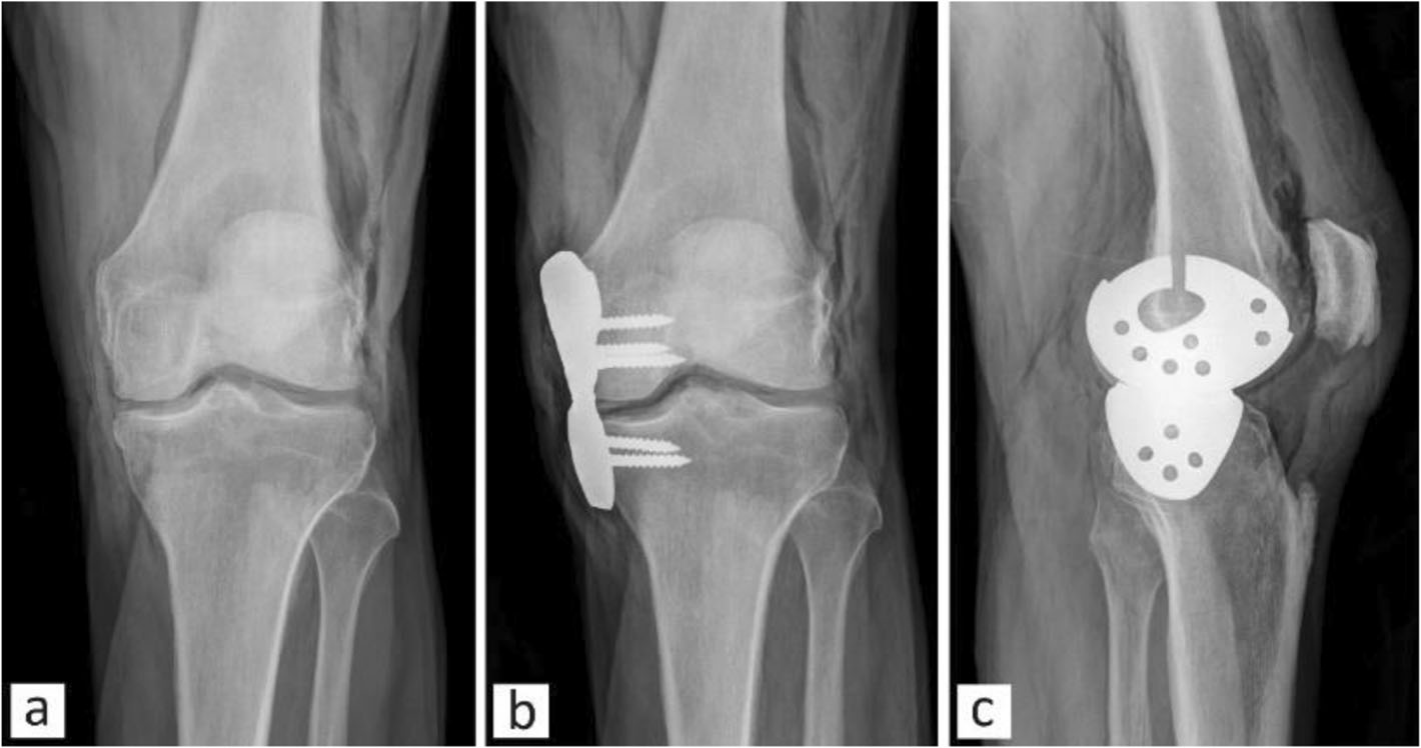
X-ray of the knee joint: a AP without implant, b AP with implant, c lateral with implant
